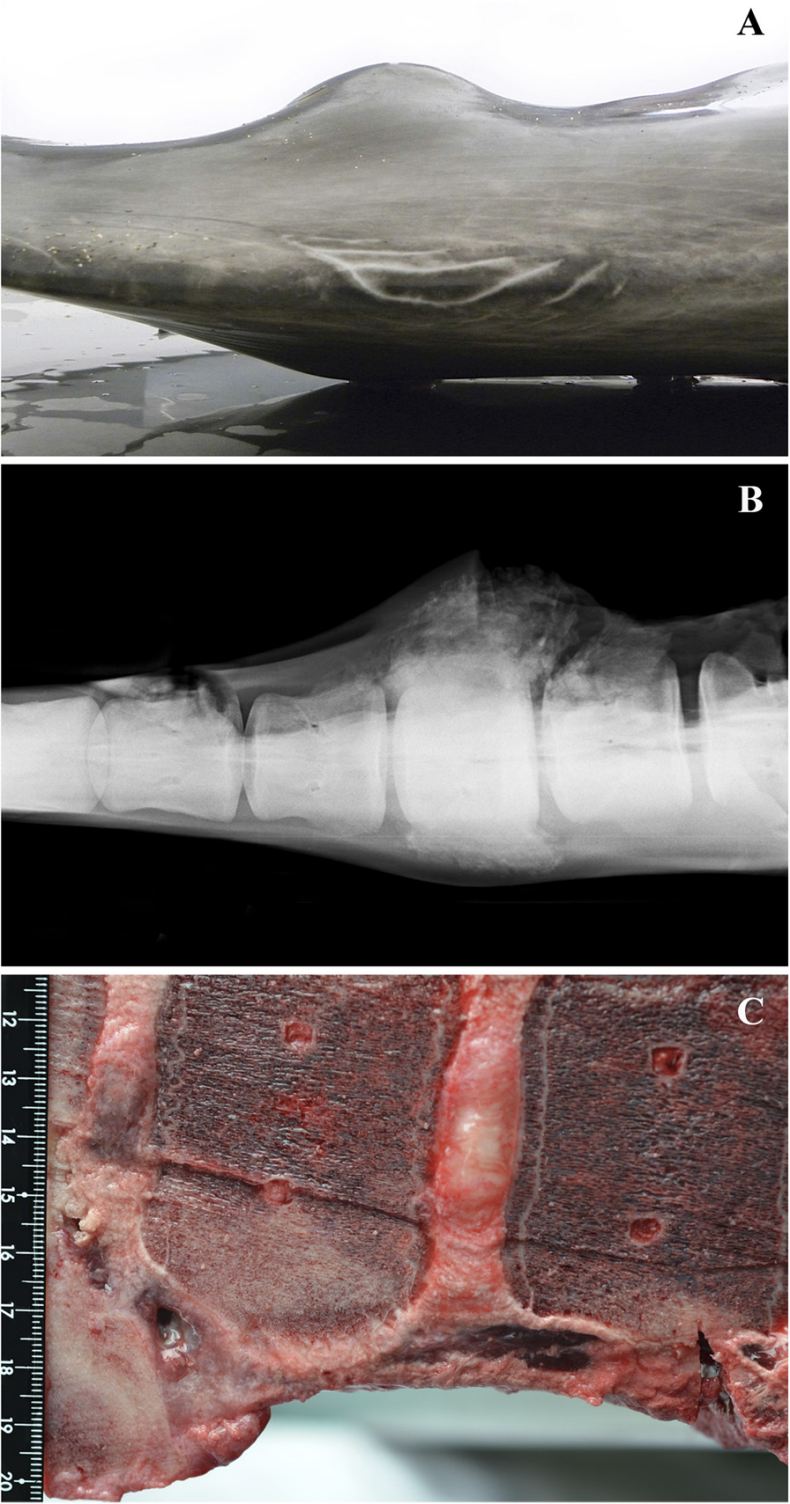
Bottlenose dolphin, case N-275/12. A. Mass in the caudal peduncle, dorso-ventral view of the lesion at necropsy. B. Radiograph from the lesion in dorso-ventral view, showing periostal proliferation with ankylosis of coccygeal vertebrae. C. Longitudinal section of the affected vertebra, showing bony proliferation at the ventrolateral side of the vertebral body, leading to ankylosis. There is formation of small abscesses. *Brucella ceti* was cultured from one of the abscesses.
